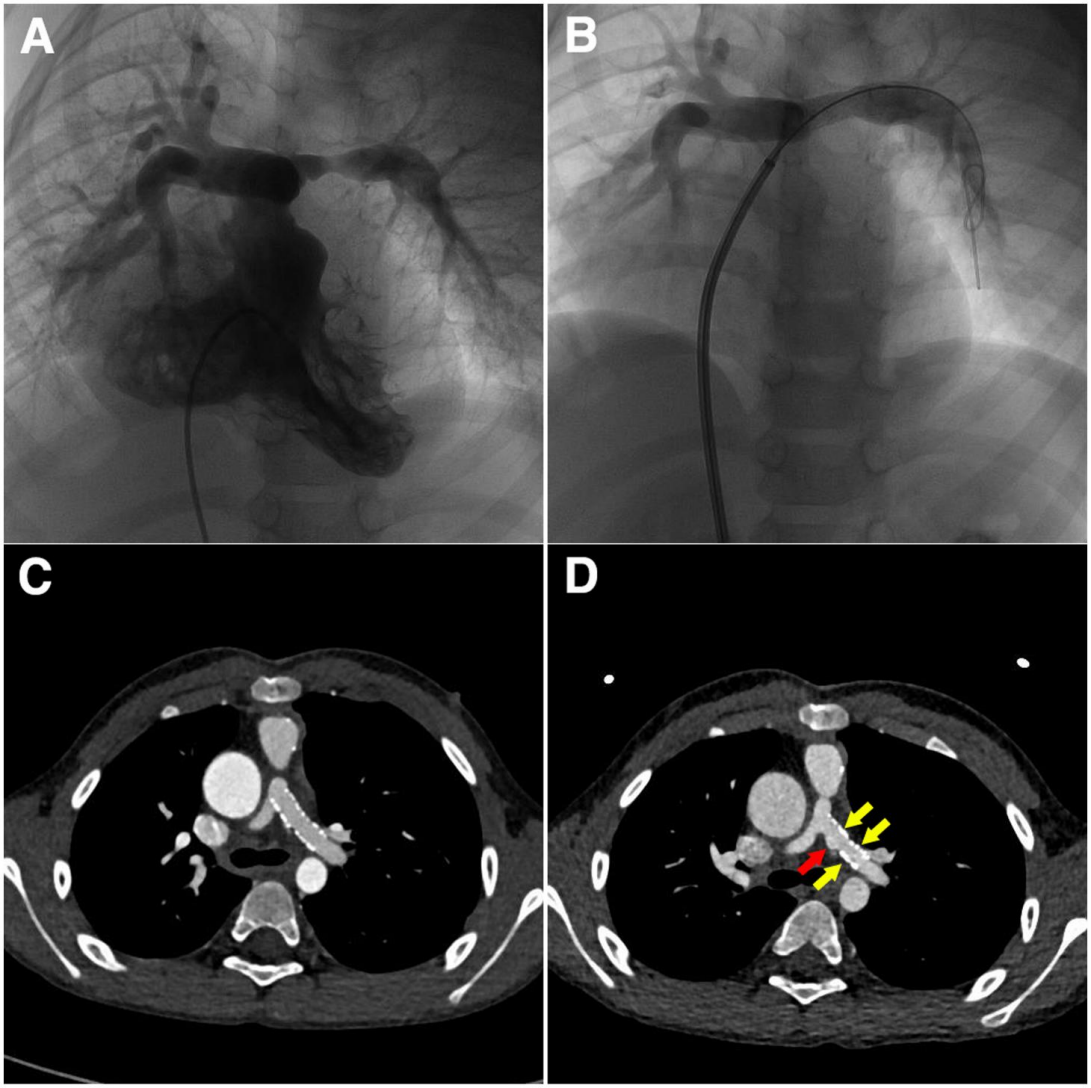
First-in-human implantation of the BBS. (A) DSA image before the procedure. (B) DSA image after the BBS implantation. (C) CT image at 1-year follow-up. (D) CT image at 2-year follow-up.
